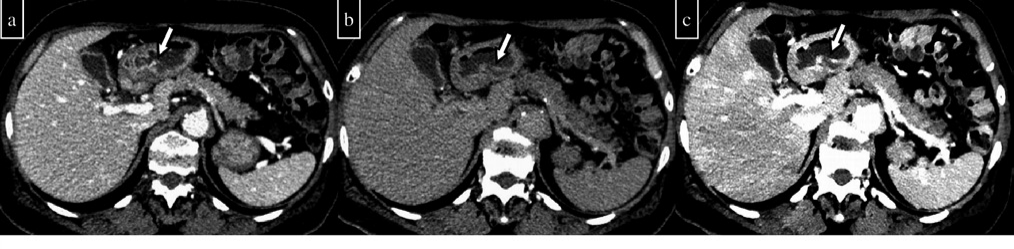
A 62-year-old woman presented with complaints of black stools and hypotension to the hospital. A contrast-enhanced two-phase (portal venous phase and delayed phase six minutes later) CT was performed to assess the cause of bleeding. (a) In the portal venous phase, a punctate high-density area was found near the gastric pylorus (arrow). (b) A punctate high-density area is seen near the gastric pylorus in the delayed phase, and the high-density area is slightly enlarged compared with that in the portal venous phase. This is considered an active haemorrhage. (c) The VMI (40 keV) of the delayed phase shows a clear spread of contrast agent compared with the original image. VMI, virtual monochromatic image.
